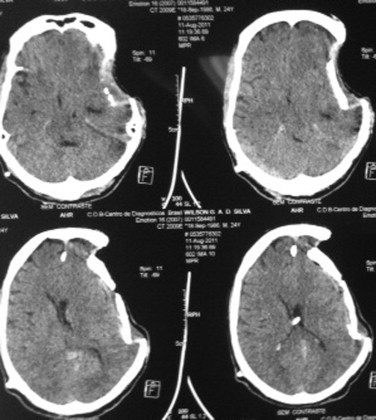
Patients CT scan exam after worsening in neurological condition.
